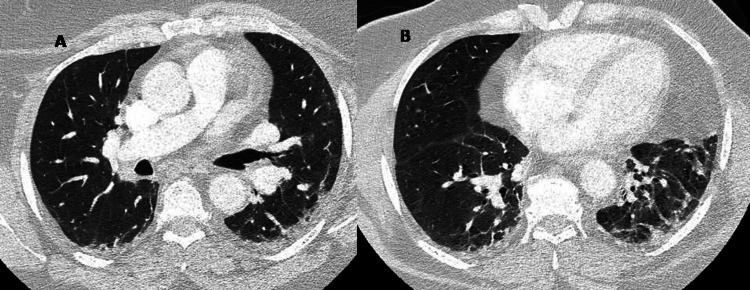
CT of the chest revealed (A) a non-specific interstitial pneumonia pattern characterized by diffuse ground glass opacities with associated reticular opacities and (B) lung architectural distortion. CT: computed tomography.
